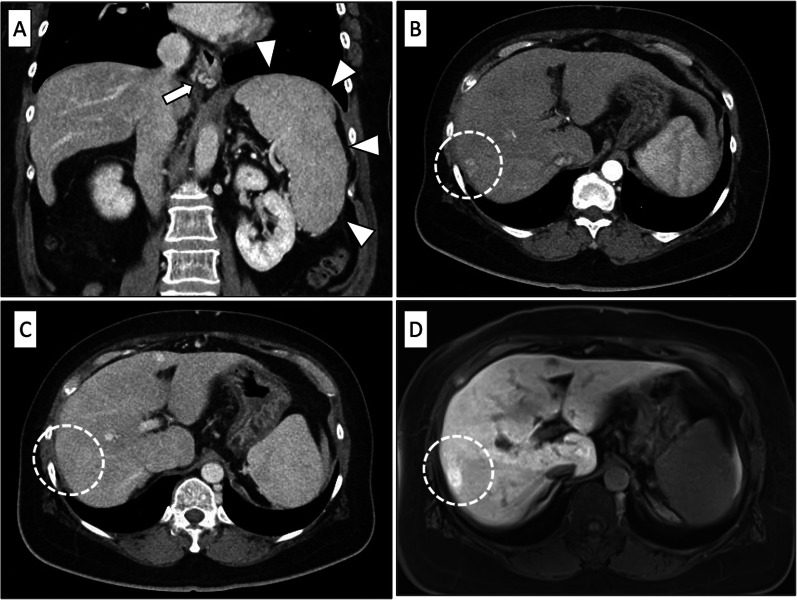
A Contrast-enhanced computed tomography showing esophageal varices (arrow) and splenomegaly (arrowheads). B Contrast-enhanced computed tomography of early phase showing a mass lesion with early enhancement (circle). C Contrast-enhanced computed tomography of portal phase showing a mass lesion without contrast agent wash out (circle). D EOB- magnetic resonance imaging of hepatocellular phase showing a mass lesion without contrast agent wash out (circle)
